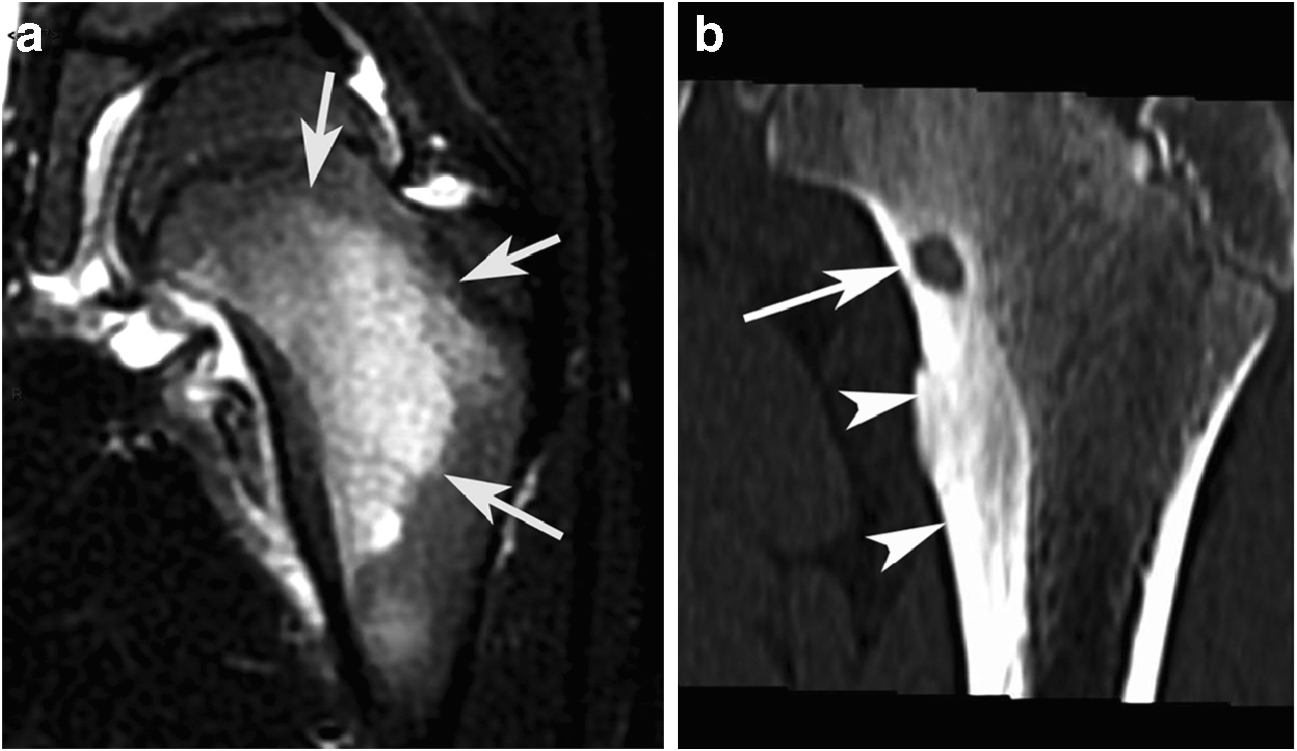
OO mimicker of stress fracture on MRI. Coronal fat-saturated T2-weighted image (a) shows a semicircular medullary edema-like pattern (arrows) adjacent to the medial cortex of the femoral neck, a frequent location for stress injuries. But a coronal CT image (b) reveals a typical OO nidus (arrow). Note important hyperostosis (arrowheads) below the OO but not on the femoral neck
